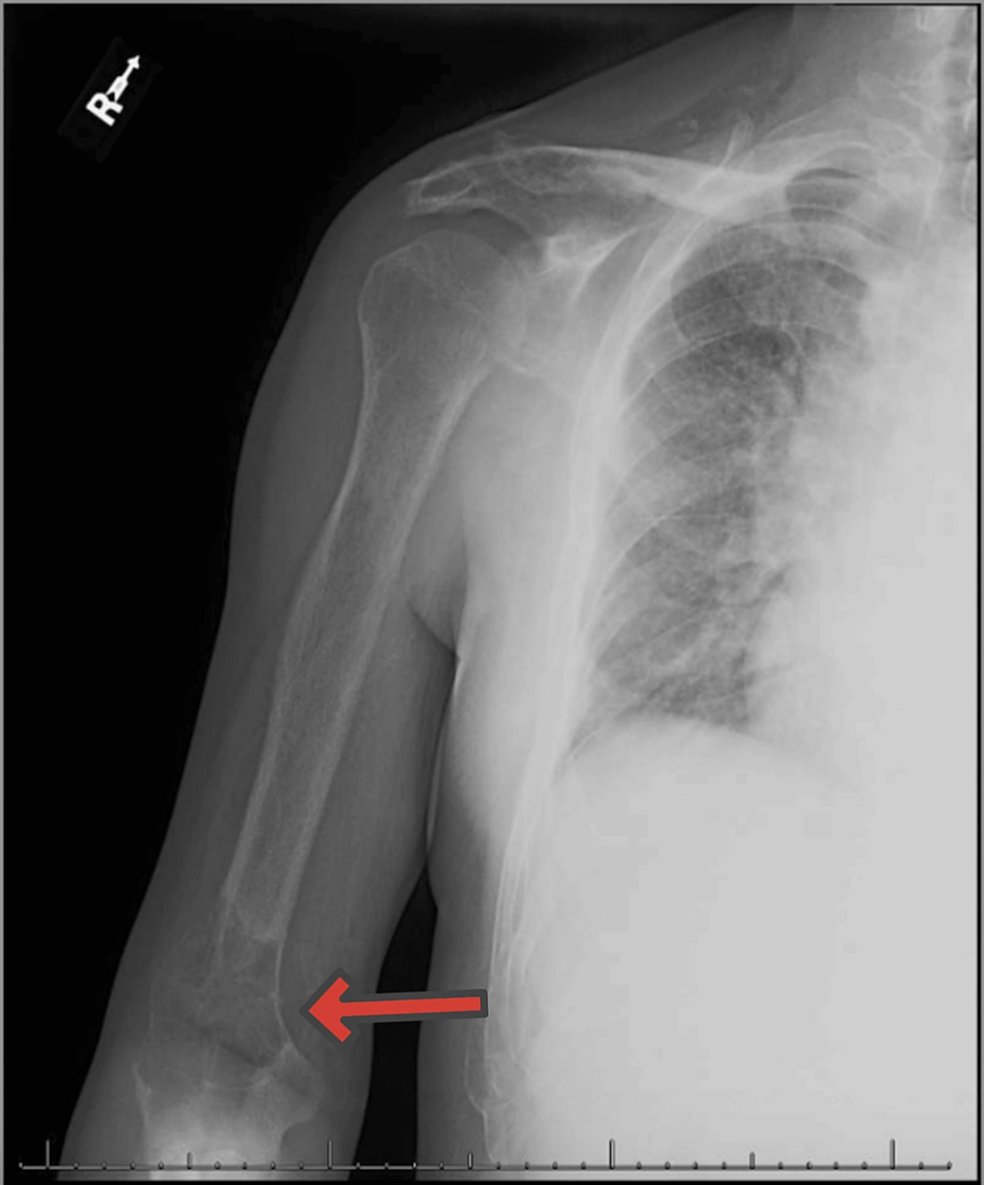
Two-view right humerus X-ray: 8-cm destructive process in the distal meta-diaphysis of the humerus with pathologic fracture, cortical-based focus on the proximal ulna, olecranon enthesopathy, and advanced vascular calcification (arrow)
